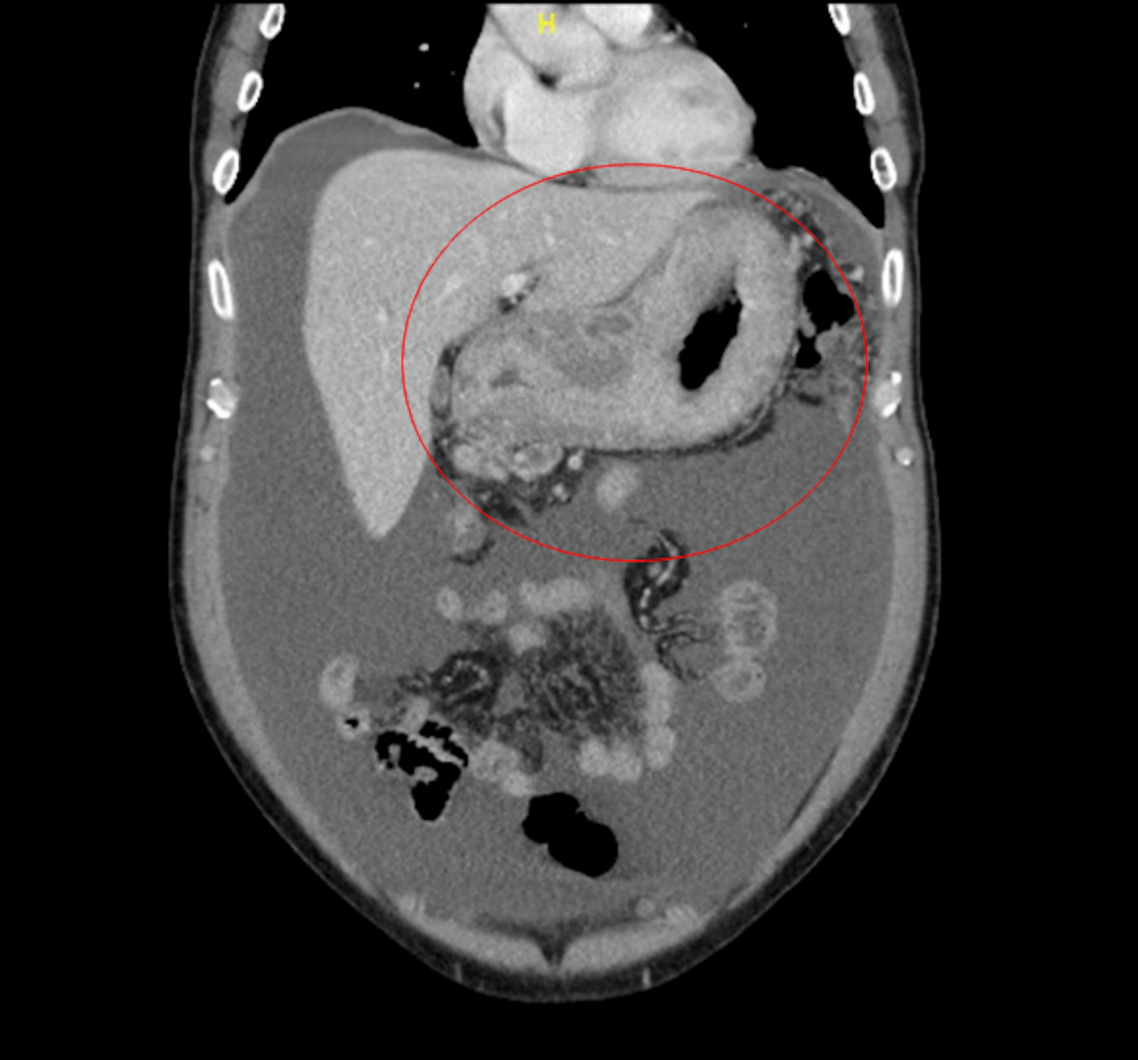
CT Abdomen and Pelvis (coronal view) demonstrating significant gastric wall thickening and ascites.
